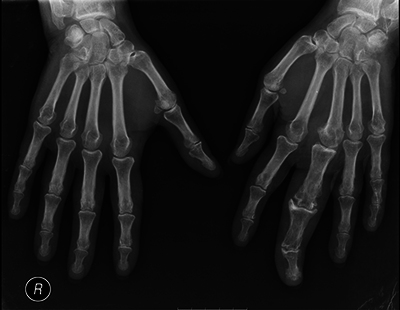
Hand radiograph, anterior–posterior projection, showing soft tissue swelling and skeletal hypertrophy affecting the 3rd digit of the left hand.
